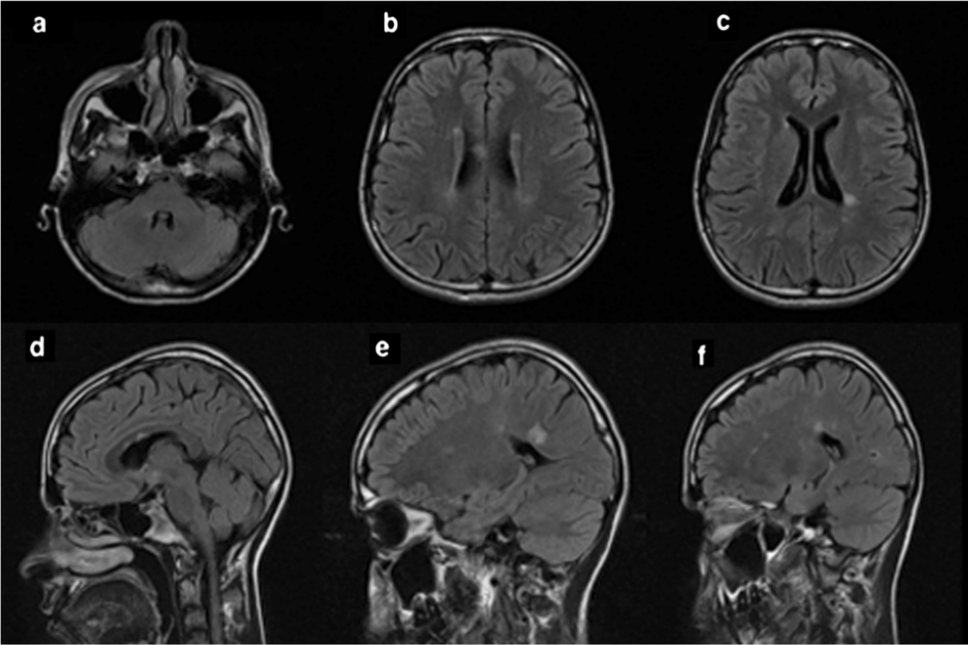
Baseline axial and sagittal T2/flair MRI brain images (September 2011) showed demyelinating plaques involving the right middle cerebellar peduncles (a), periventricular areas (b,c) and corpus callosum (d-f).
